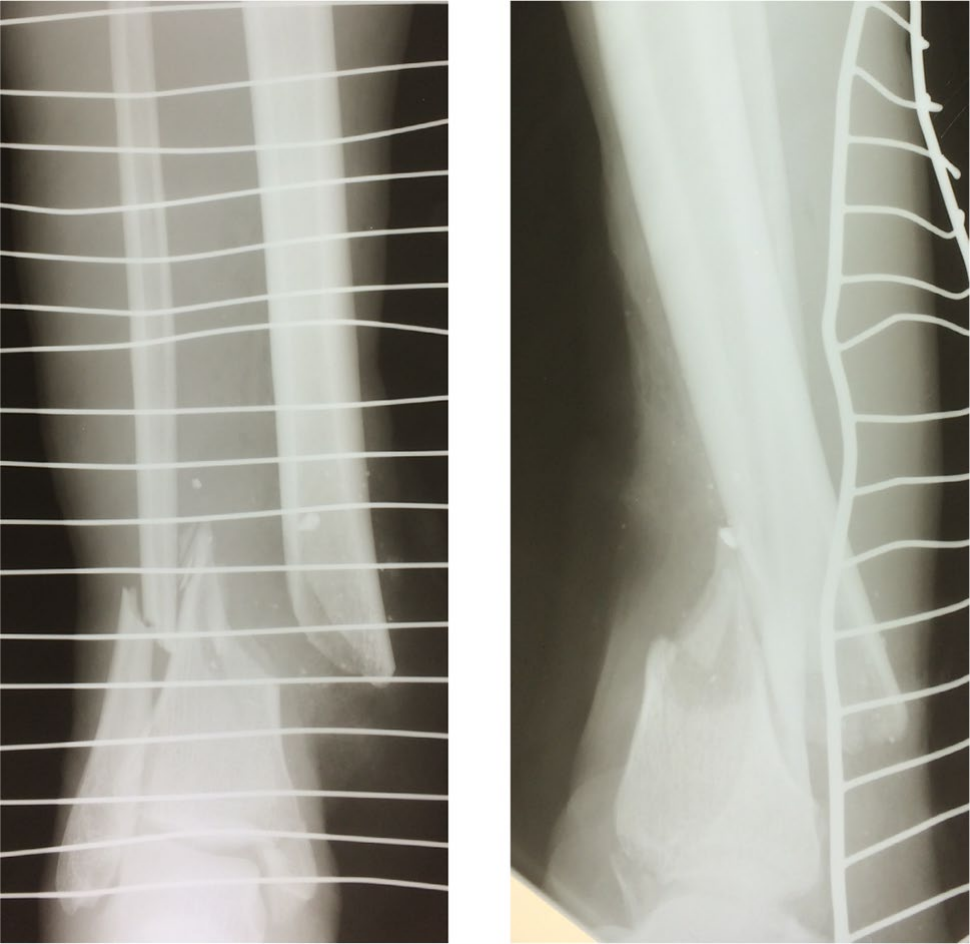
X-ray in two planes of a patient (45year-old male) with a right tibial shaft fracture 42 A2.3 III° B open
